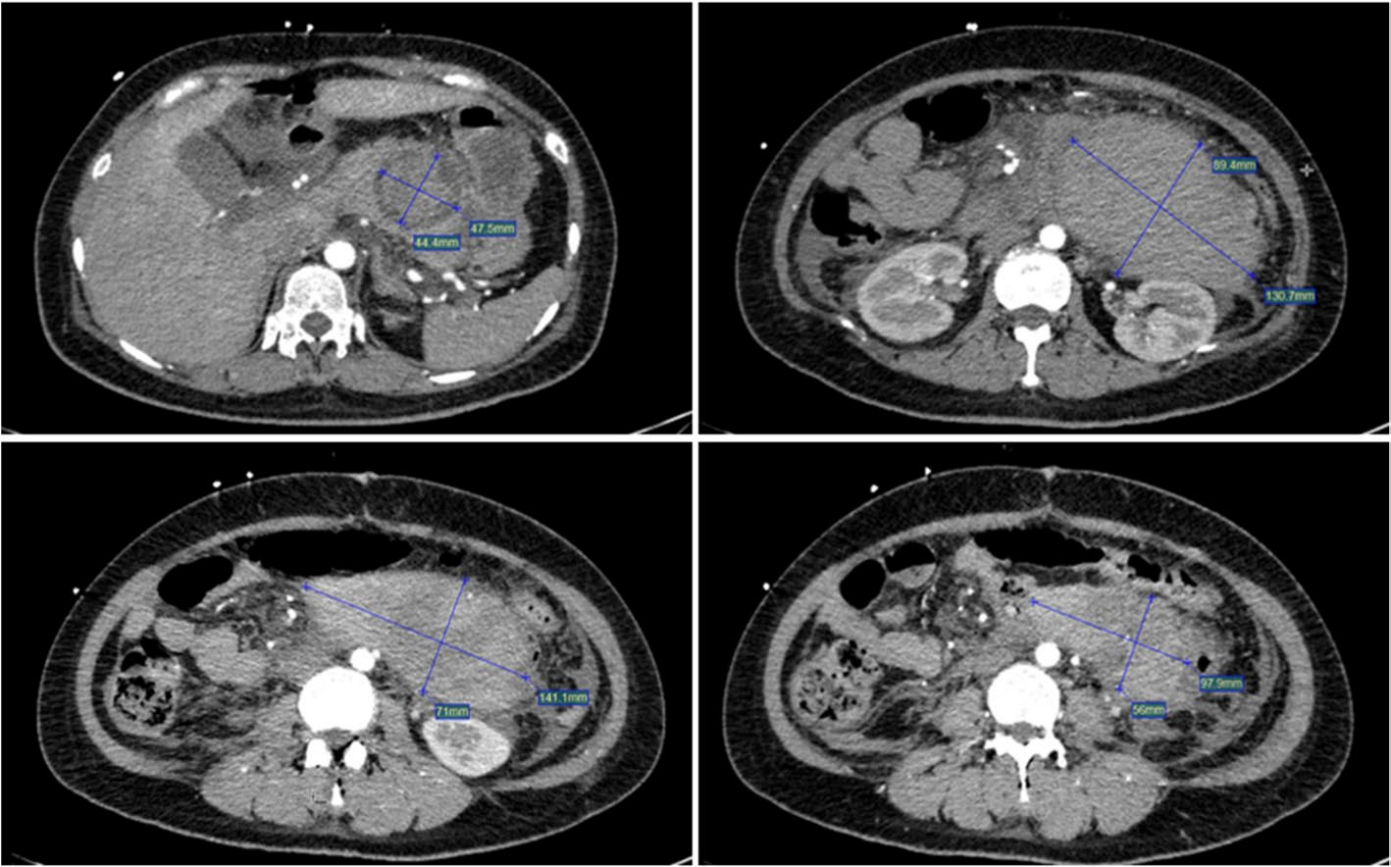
Preoperative CTA showed a huge RPH without a clear target vessel. Maximum cross-sectional area is approximately 13 cm × 8.9 cm. Average CT value: 67Hu
